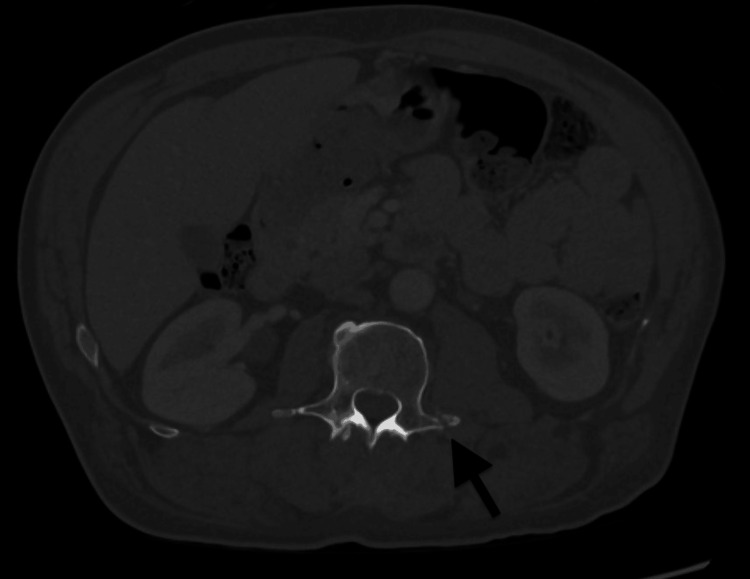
CT of the abdomen (axial view) revealing displaced non-acute appearing fractures of the left L2 and L3 transverse processes.
